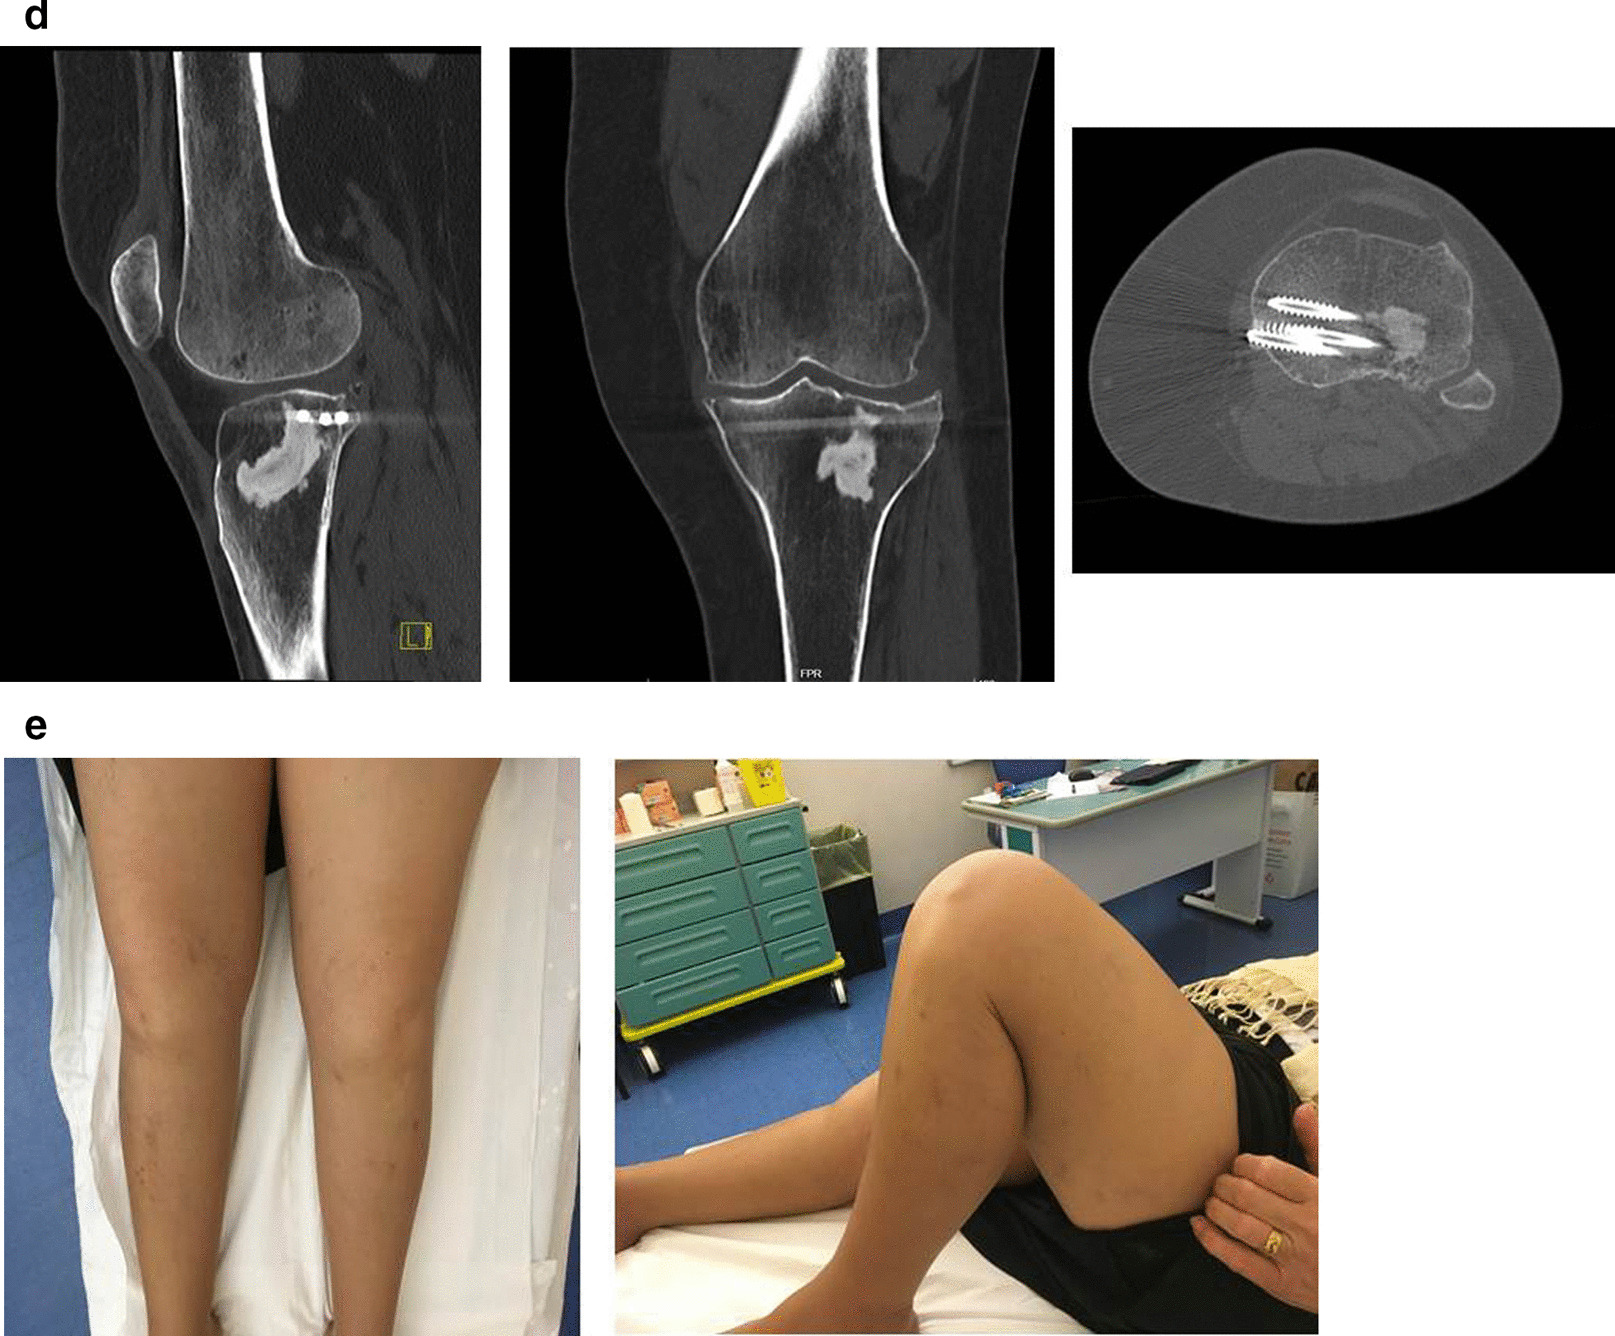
A 73-year-old woman. A Preoperative X-ray and CT scan demonstrating Schatzker type III fracture. B Postoperative X-ray: anatomic reduction of articular surface and Cal-Cemex. C Six-month postoperative X-ray: no significant change in bone–cement interface or loss of reduction, good bone–cement interdigitation. D Six-year postoperative CT scan demonstrating osteointegration and no loss of reduction. E Six-year clinical evaluation: good axis and range of motion
